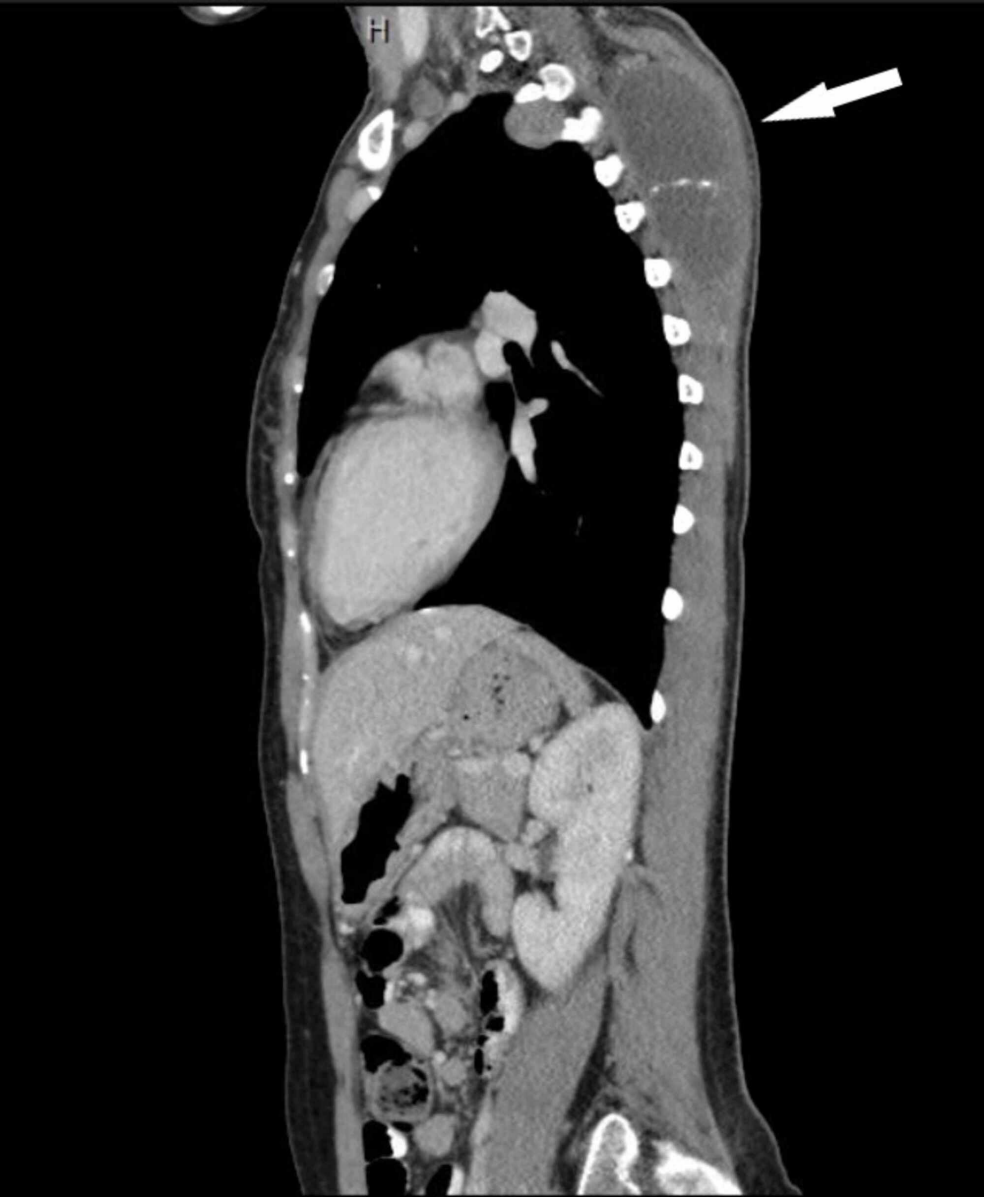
CT scan of sagittal section showing left trapezius muscle mass (white arrow)
